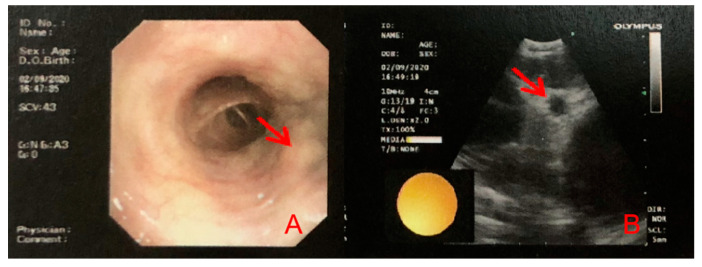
(A) Bronchoscopy shows multiple small nodules in the mucosa of the distal left main bronchus and the right middle bronchus. (B) Endobronchial ultrasonography shows enlargement of lymph nodes in 4L, 4R, 7, 11L, and 11R groups.
